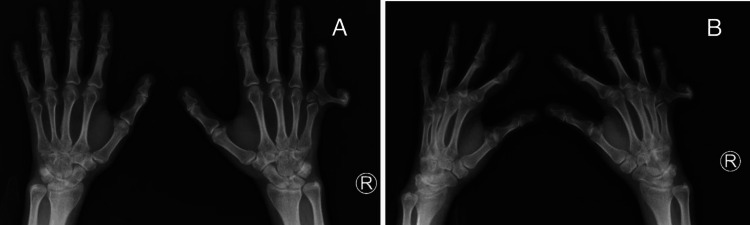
X-ray images of both hands of the patient show type 2 post-axial polydactyly with delta phalanx of the right hand; A. anteroposterior view; B. oblique view
